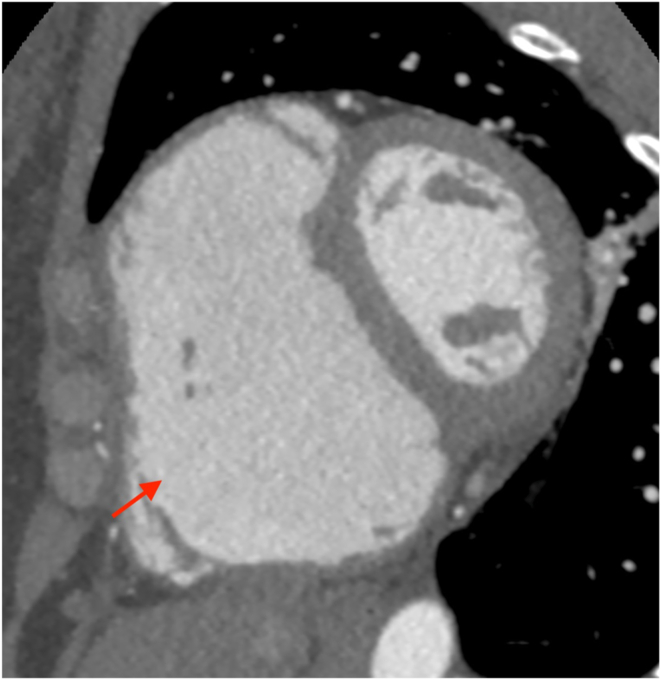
Cardiac computed tomography, oblique sagittal plane, cardiac mid-LV short-axis view, demonstrates a dilated RV *(arrow)* with abnormal septal position consistent with right ventricular volume-overload.
